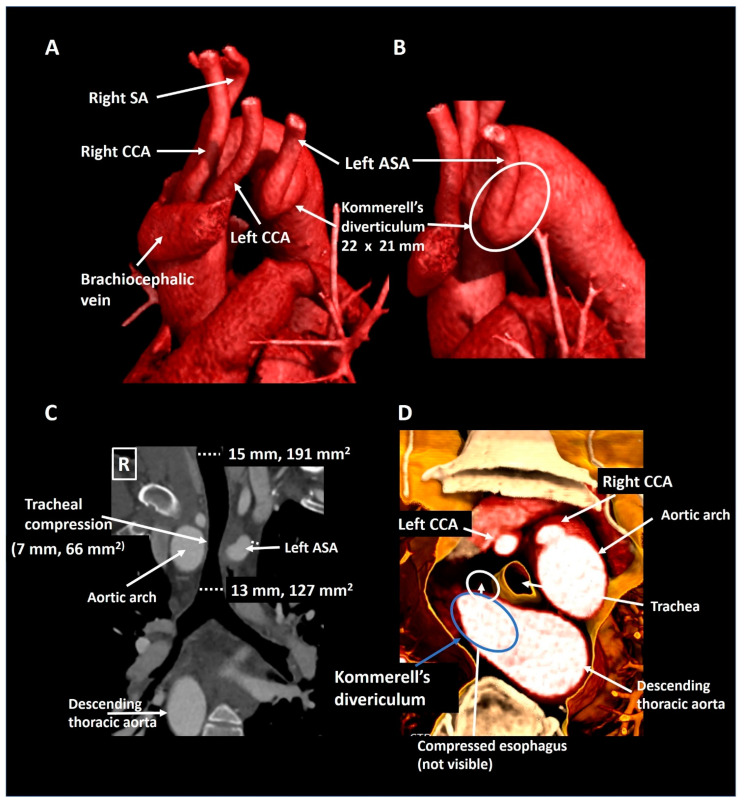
Patient 2. Panel (A) shows a lateral computed tomography reconstruction depicting a right-sided aortic arch with an aberrant left subclavian artery. The zoomed posterolateral reconstruction in panel (B) shows that the aberrant left subclavian artery is arising from a Kommerell’s diverticulum. Panel (C) is a coronal reconstruction that reveals tracheal compression. The axial 3D cross-section (panel (D)) shows partial posterior compression of the trachea which was secondary to tracheal bowing and not directly to compression by the vascular structures. ASA, aberrant subclavian artery; CCA, common carotid artery; SA, subclavian artery.
